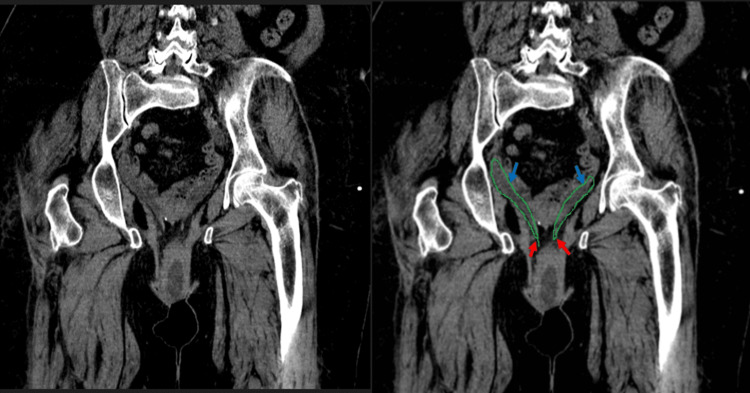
Coronal CT scan of the abdomen showing hydronephrosis caused by external compression. Original and outlined coronal abdominal CT scan without intravenous contrast displaying both ureters (outlined by the green line), with notable dilation (indicated by blue arrows) along with tapering constriction of the distal bilateral segments (highlighted by red arrows), suggestive of external compression.
